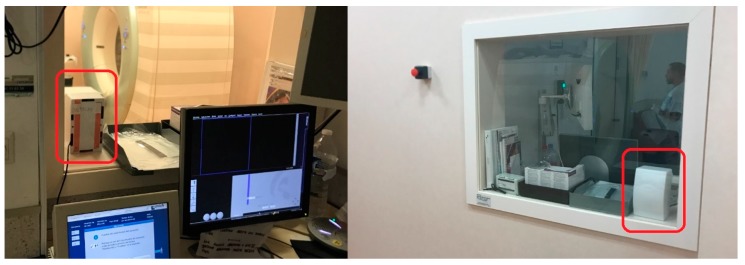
Device placed next to the CT room.
